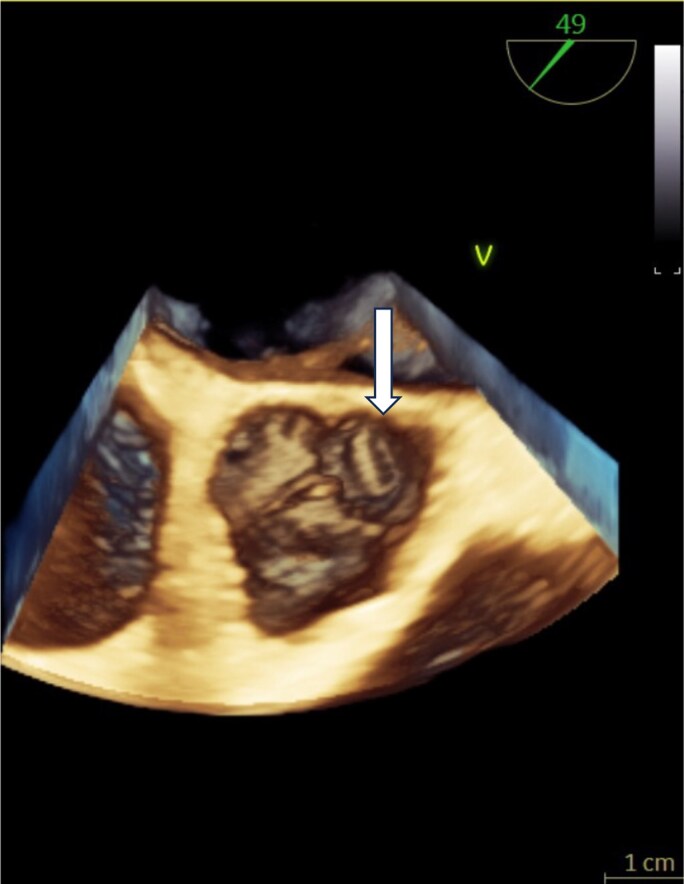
Three-dimensional transesophageal echocardiography. En-face 3D view clearly delineating perforation of the left coronary cusp, providing structural confirmation of the anatomical defect responsible for acute severe aortic regurgitation.
